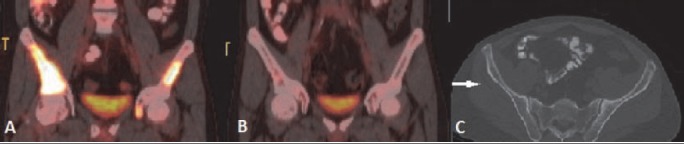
NHL involving bone: (A) PET/CT image shows FDG avid deposit in right ilium. (B) Post chemotherapy complete metabolic response is seen in repeat PET/CT scan. (C) CT scan shows no significant change except minimal cortical irregularity (arrow)
